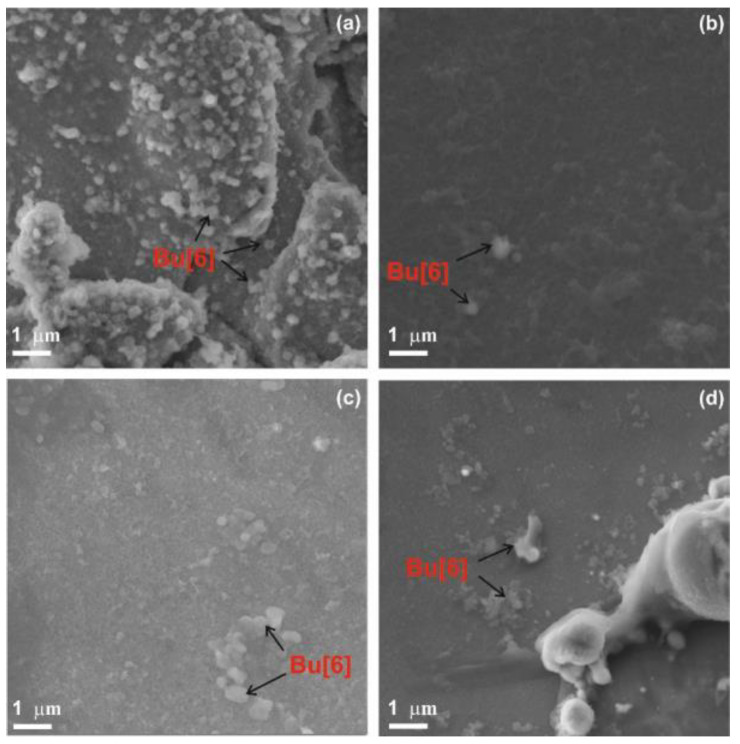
SEM images of the surface of porous TiNi with bambusuril deposited under vacuum (a); without external effects (b); under microwave exposure (c); under ultrasonic exposure (d).
